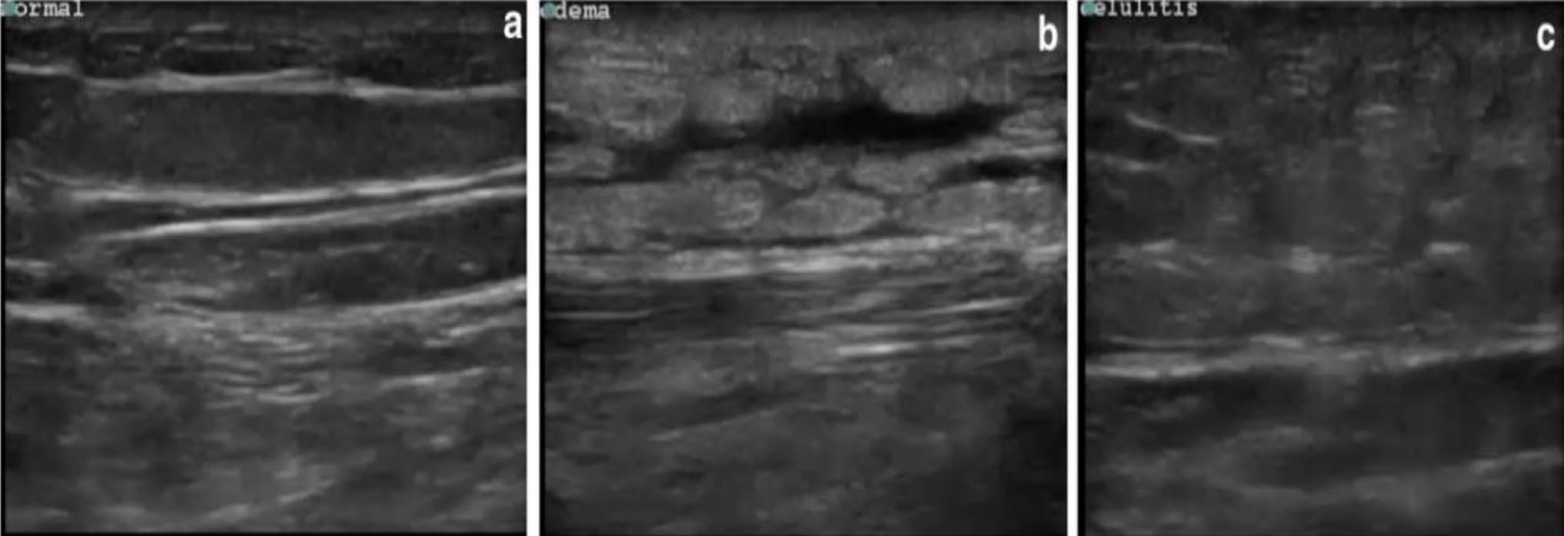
Differential diagnosis to be considered from ultrasonography findings of subcutaneous thickening and fascial fluid. a Normal soft tissue, b peripheral edema, and c cellulitis using a linear probe. Source: Magalhaes et al. with permission
